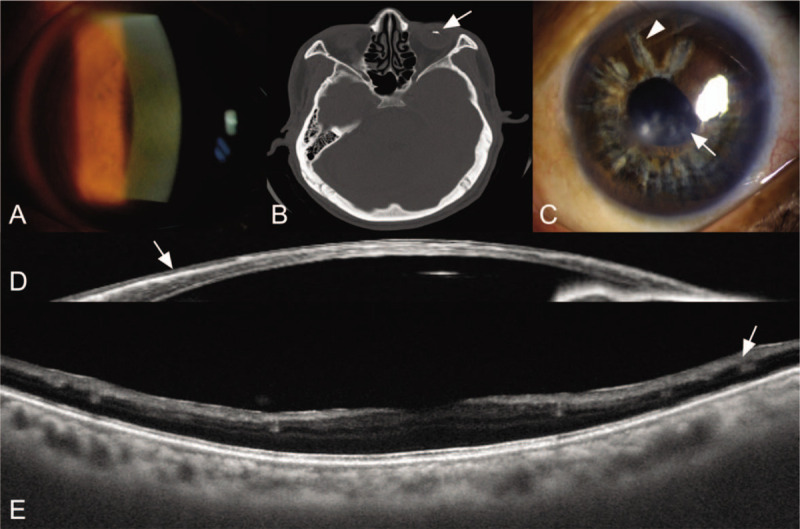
Ocular siderosis at initial visit and 6 years after removal of intraocular foreign body. (A) A slit lamp photograph at initial visit showing rust-colored hue visible on corneal stroma. (B) Orbital computed tomography showing intraocular foreign body (arrow) in the vitreous. (C) A slit lamp photograph at 6 years after removal of intraocular foreign body showing corneal haziness (arrow) and iris heterochromia (arrow head) resembling spokes of a wheel. (D) Anterior segment optical coherence tomography of ocular siderosis showing linear hyperreflectivity in the anterior corneal stroma (arrow). (E) Posterior segment optical coherence tomography of ocular siderosis showing degeneration of the inner retina (arrow).
